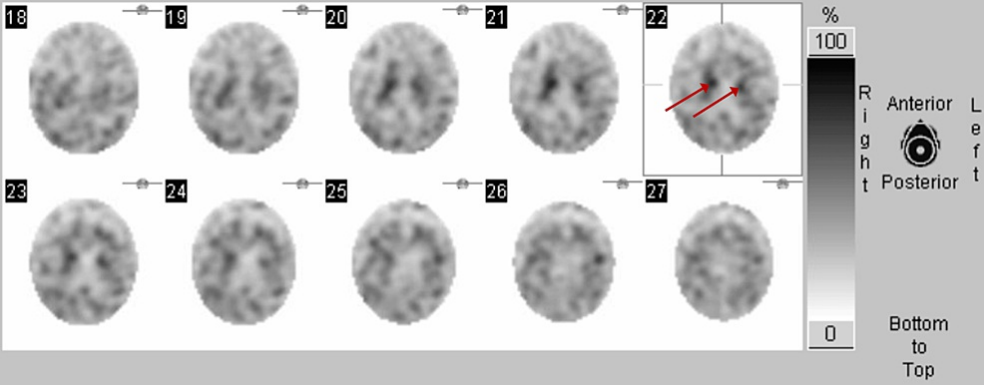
Axial images of (I-123)-FP-CIT DaT-SPECT Images show bilaterally absent putamen binding and heterogenous reduced binding in the left caudate nucleus more than the right. Red arrows indicate bilateral caudate nuclei. DaT-SPECT: (I-123)-FP-CIT single-photon emission computed tomography
